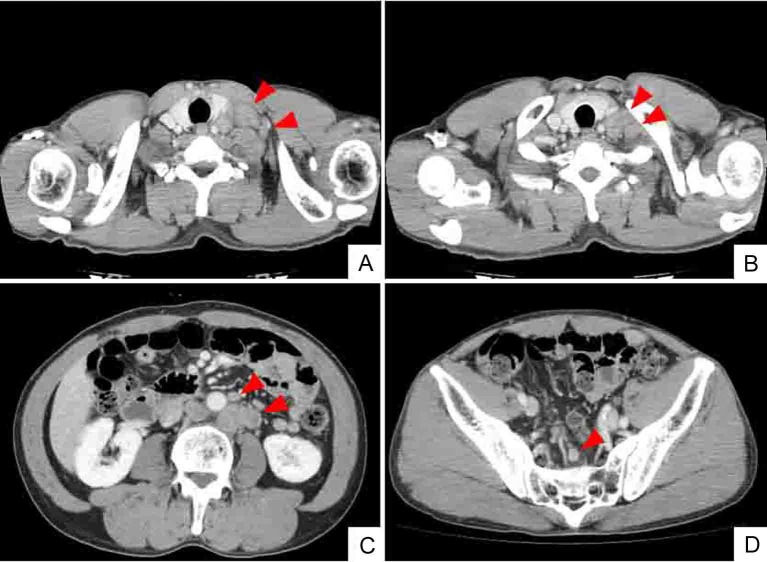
Whole body CT (computed tomography) shows mass of swollen left cervical (A) and supraclavicular (B) LNs. CT findings also indicate enlarged abdominal periaortic, inferior mesenteric and surrounding (C), and colic (D) LNs (arrowheads). However, CT findings do not indicate sigmoid colon tumor.
